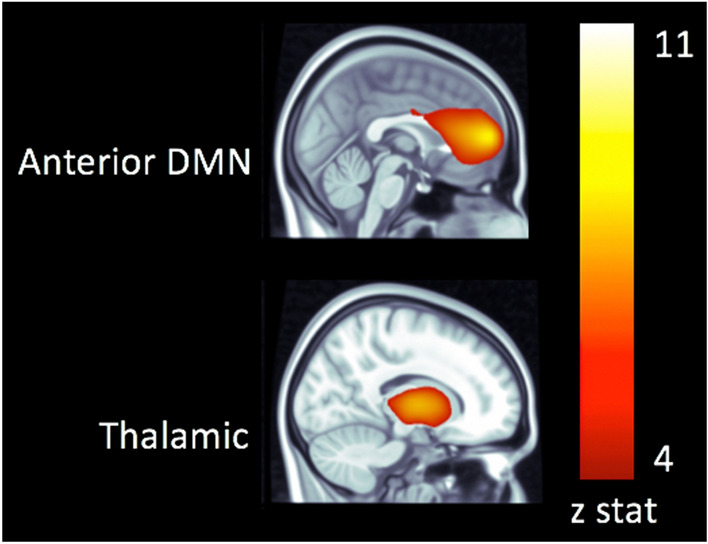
Resting state networks overlaid on a template MRI in the space of the Montreal Neurological Institute.
